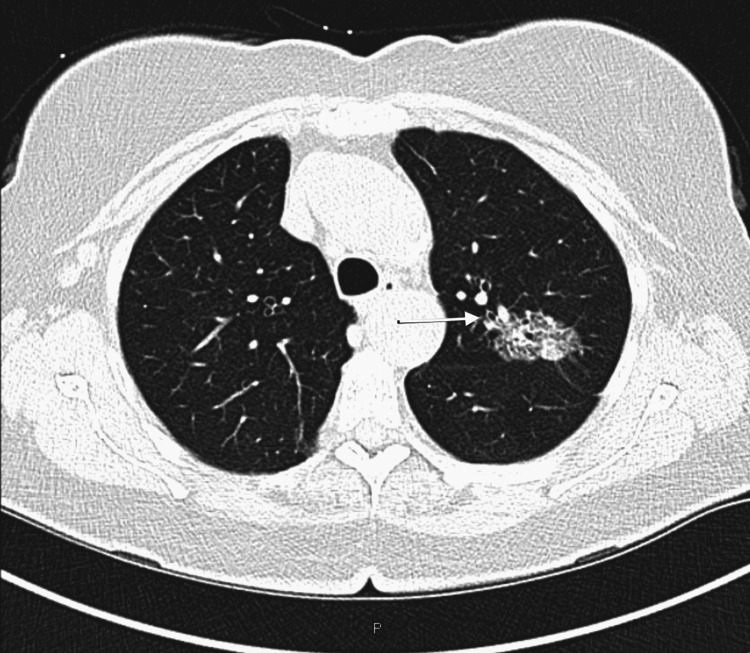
CT chest in the axial window shows a solid mass in the left upper lobe measuring 4.3 x 2 cm in axial dimensions and 3.9 cm in craniocaudal dimensions. The solid component measures 2.7 x 1.6 cm in axial dimensions and 1.7 cm in craniocaudal dimensions.
